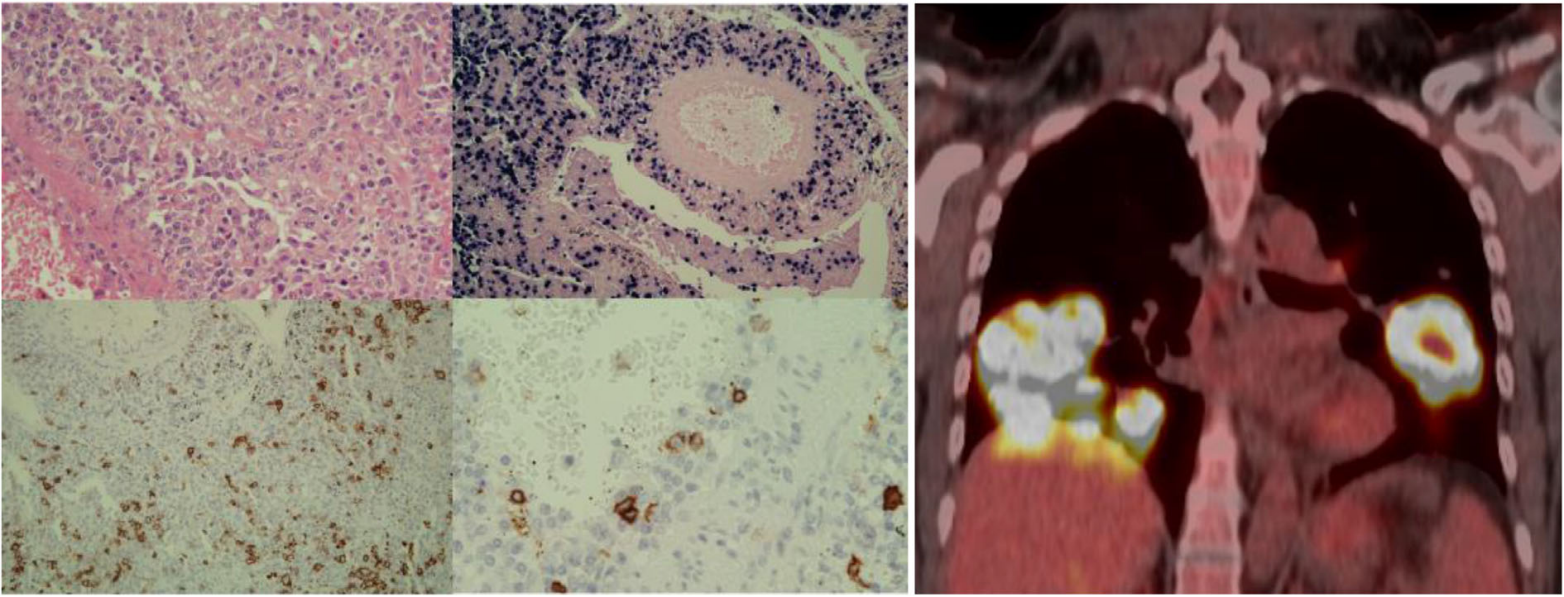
Diagnosis was elusive, with worsening symptoms corticosteroids were commenced and biopsy planned. Ground glass infiltrate improved (on CT) with steroids, thus, while the differential for consolidation is wide, working diagnoses leaned toward lymphoproliferative disorders (LPDs), which cause the entire myriad of lung radiological appearances.
